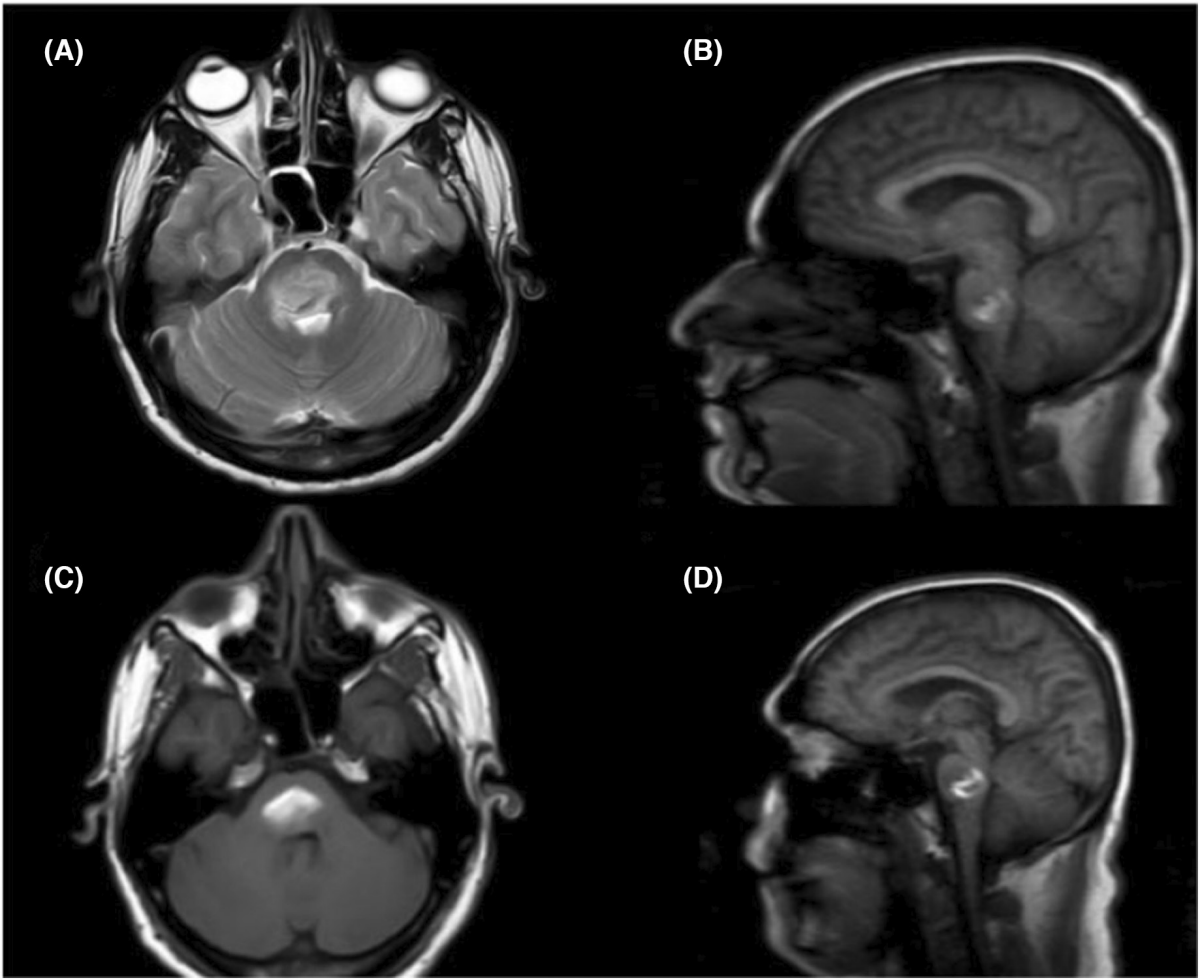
T1/T2 hyperintensities with adjacent perilesional edema are noted in pons extending to involve the right midbrain and superior part of the medulla oblongata, causing mild narrowing of the fourth ventricle. Areas of diffusion restriction and blooming were noted. Suspicious small feeding vessel noted‐ Few tiny foci of blooming noted in the bilateral lentiform nucleus and external capsule ‐ likely microhemorrhages. Mild periventricular T2/FLAIR hyperintensities noted ‐ likely vessel ischemic changes. Profile based on ICF Core Components.
